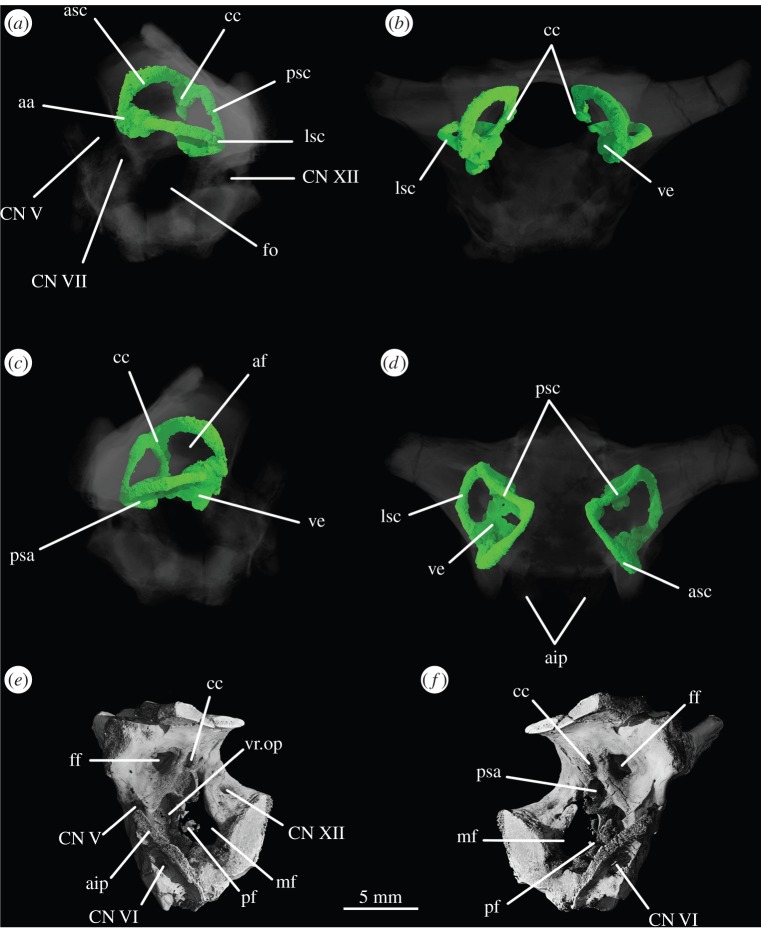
CT reconstructions of braincase of SAM-PK-7696 showing structures of inner ear in (*a*) left lateral, (*b*) anterior, (*c*) right lateral and (*d*) dorsal views, and showing (*e*) right medial wall in anteromedial view and (*f*) left medial wall in anteromedial view. In (*a*–*d*), bones of the braincase have been rendered transparent for better visualization of the suture lines. For abbreviations, see table 1.
